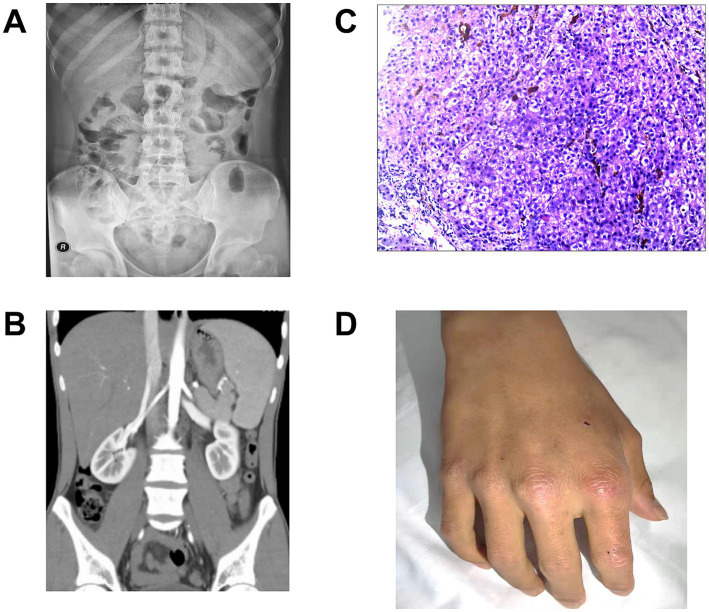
Intestinal obstruction and cholestasis. (A) Abdominal X-ray indicated the symptoms of intestinal obstruction in the lower right abdomen. (B) Contrast-enhanced abdominal CT indicated hepatosplenomegaly and excluded biliary obstruction, tumors, or portal vascular abnormalities. (C) Liver biopsy indicated active cirrhosis and severe intrahepatic cholangitis (HE, ×100). (D) Nonblistering cutaneous photosensitivity in light-exposed areas.
